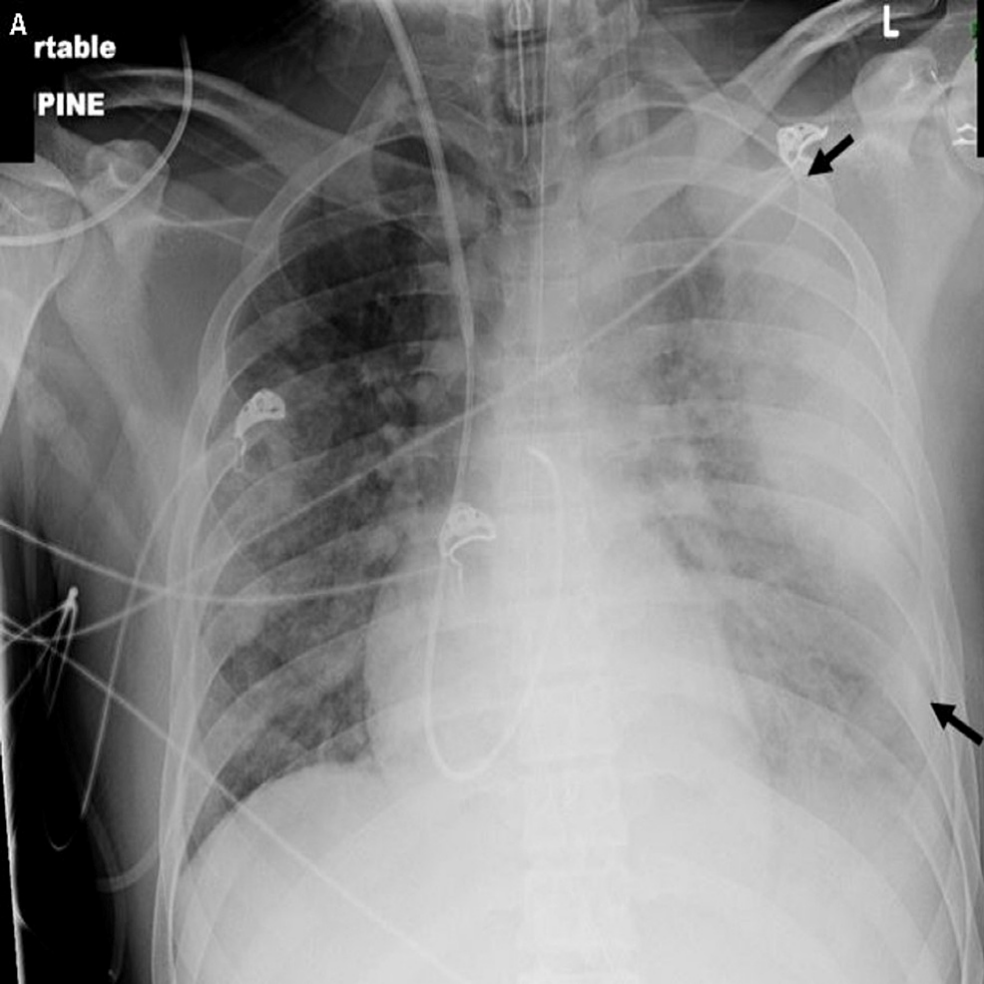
Chest X-ray AP chest radiograph demonstrates multiple nodular opacities throughout both lungs and a complex, partially loculated left pleural effusion (black arrows) AP: anteroposterior
